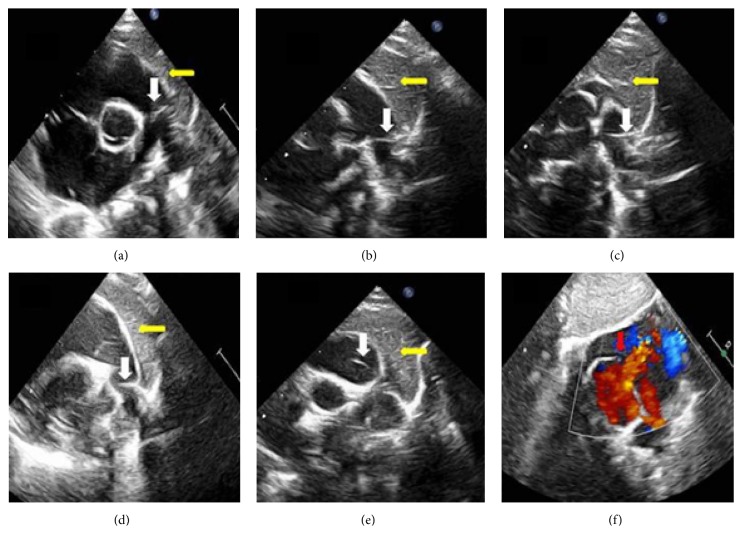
(a) Aortic short-axis view. (b, c) Left high parasternal view. (d, e) Aortic short-axis and pulmonary artery long-axis views. (f) Color Doppler imaging in the subxiphoid two-atrium view. A linear artifact was clearly seen (white arrowhead). The thymus gland was prominently seen (yellow arrowhead).
